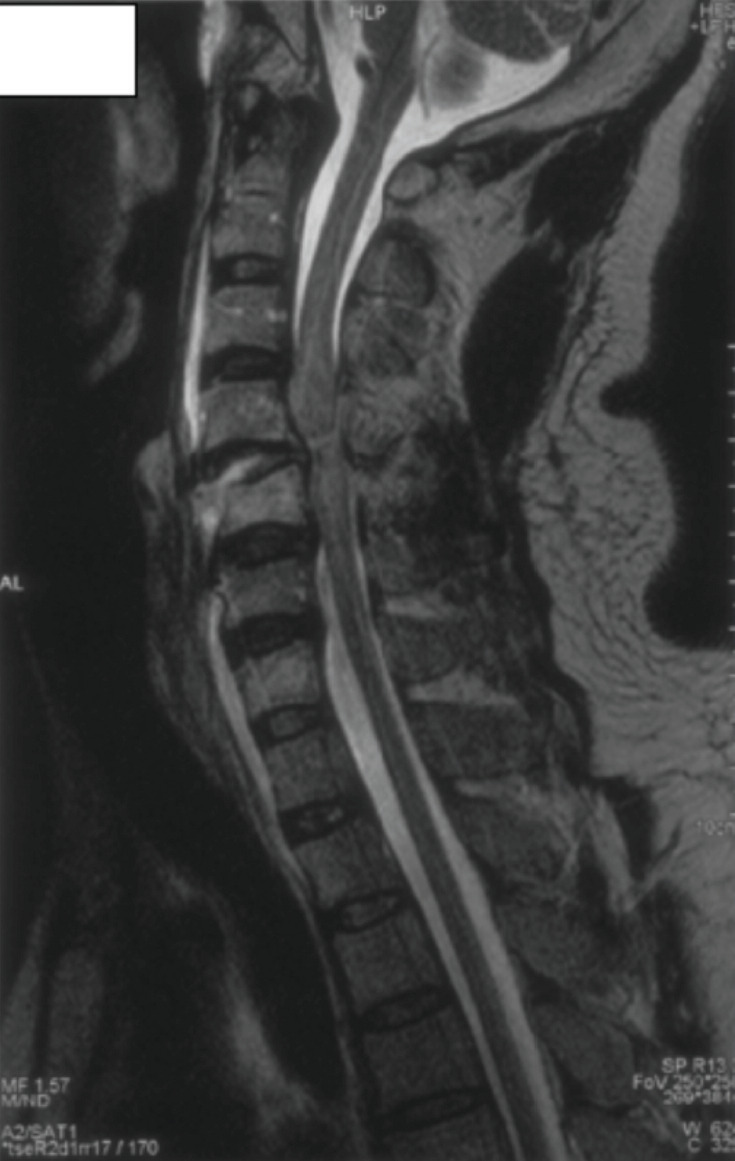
Complete transection of C5 level of spinal cord and cord oedema (Day 1 MRI after admission).
